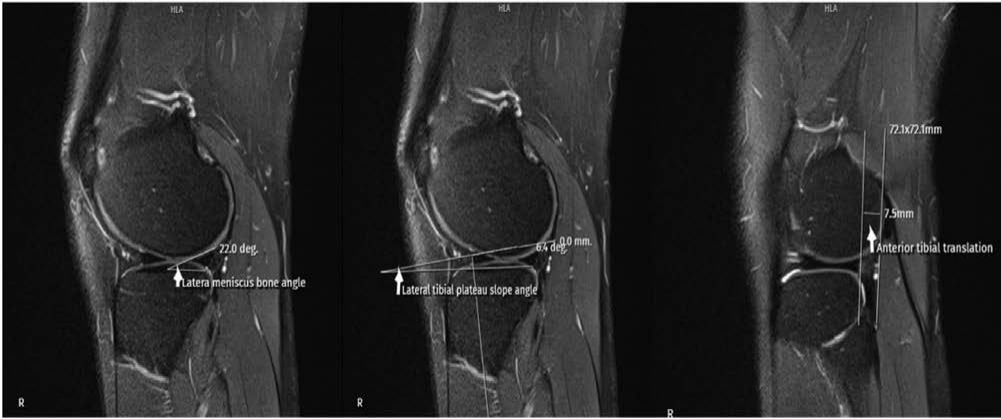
MRI measurement techniques of LMBA, LTPS, and ATT. ATT, anterior tibial translation; LMBA, lateral meniscus bone angle; LTPS, lateral tibial plateau slope; MRI, magnetic resonance imaging.
